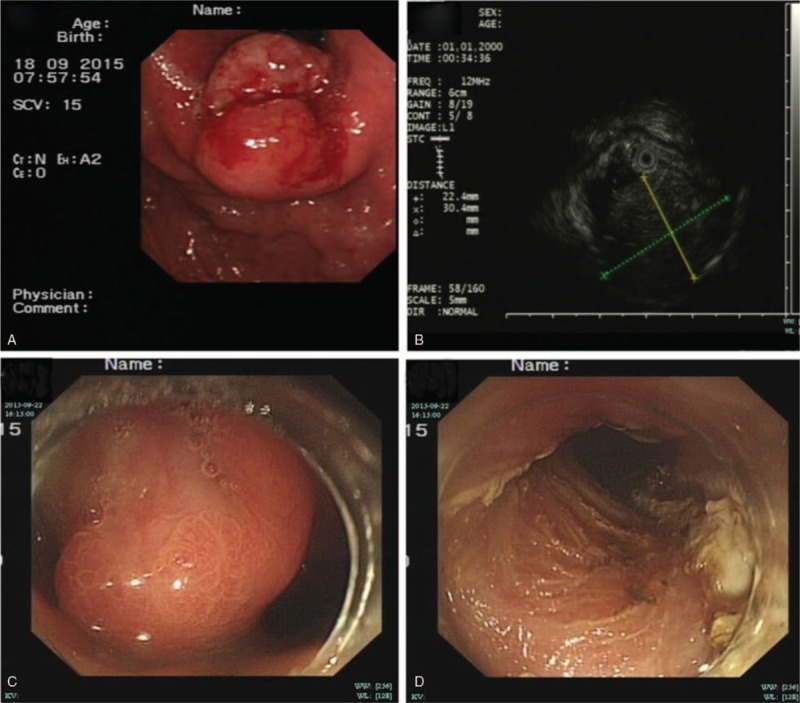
The preoperative (A, B) and intraoperative (C, D) endoscopy showing the tumor. (A)+(B): Endoscopic ultrasound showing a hypoechoic tumor located in the gastroesophageal junction with the size of 22.4 mm × 30.4 mm; (C): Intraoperative normal white light of the tumor (D): The Resected surface after excision.
